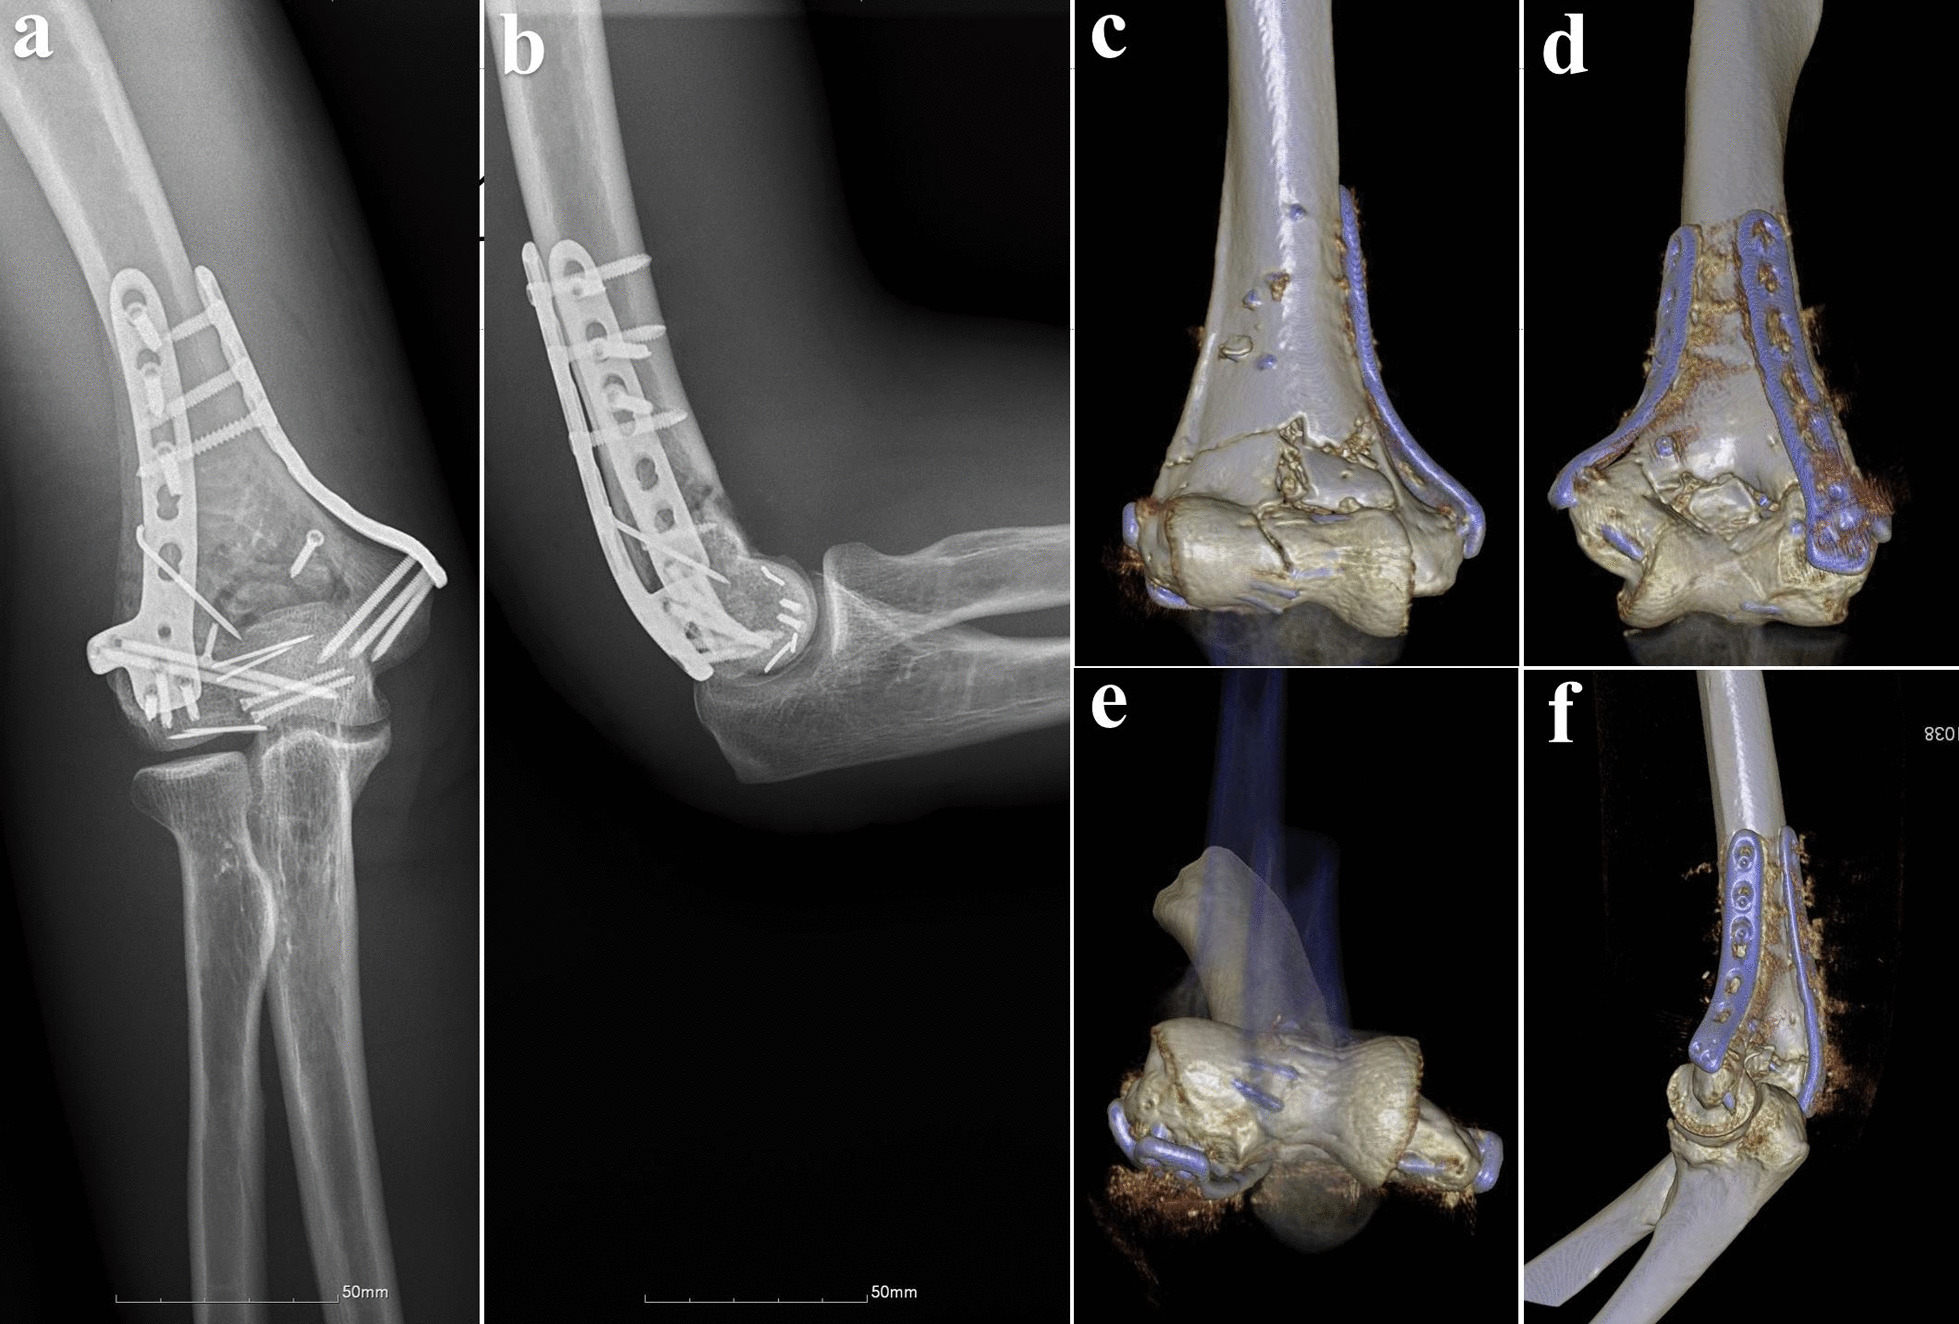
The postoperative radiographs were shown during the follow-up. a–d Postoperative X-Rays and postoperative reconstructed 3D CT images of AO type13C3 distal humerus fracture
